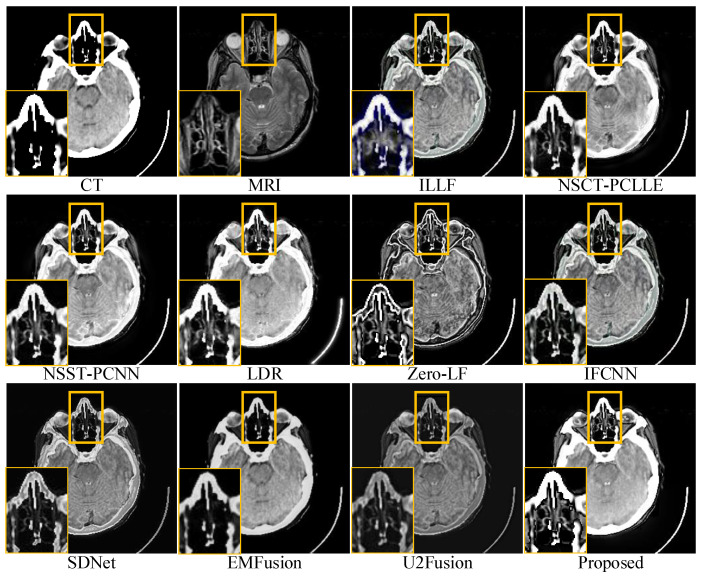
Comparison of performances of various methods on computed tomography magnetic resonance imaging (CT-MRI) source images. For a clear comparison, we select a same region (i.e., the yellow box) in each image and zoom in it in the bottom left corner.
